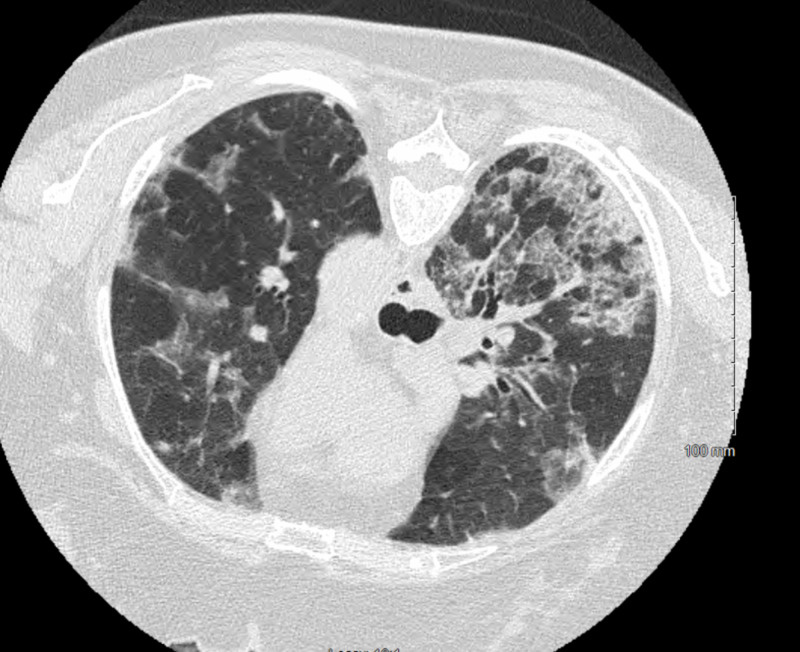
CT axial section demonstrating multifocal moderate geographic ground glass opacities bilaterally, distribution relatively diffuse with a peripheral predominance
